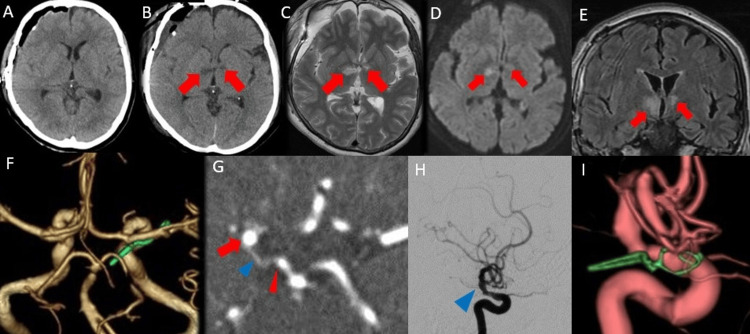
Postoperative imaging findings. (A) Non-contrast computed tomography (CT) obtained on postoperative day one, axial view, showing no apparent abnormality in the brain parenchyma. (B) Non-contrast CT obtained on postoperative day three, axial view, demonstrating low-density areas in the bilateral ventral thalami (arrows). (C) T2-weighted magnetic resonance imaging obtained on postoperative day 11, axial view, demonstrating infarcts in the same bilateral ventral thalamic regions. (D) Diffusion-weighted imaging obtained on postoperative day 11, demonstrating bilateral diffusion-restricted lesions corresponding to the abnormalities seen on the T2-weighted and fluid-attenuated inversion recovery images. (E) Fluid-attenuated inversion recovery magnetic resonance imaging obtained on postoperative day 11, coronal view, demonstrating infarcts in the same bilateral ventral thalamic regions. (F) Postoperative three-dimensional computed tomography angiography (CTA), superior view, showing that the tip of the clip does not extend to the contralateral side. (G) Postoperative three-dimensional CTA, axial view, showing the posterior communicating artery (blue arrowhead) arising posteriorly from the internal carotid artery (red arrow), as in the preoperative study. The P1 segment is also well visualized (red arrowhead). (H) Digital subtraction angiography obtained on postoperative day 17, lateral view, clearly demonstrating the posterior communicating artery (blue arrowhead). (I) Three-dimensional rotational angiography reconstructed image obtained from the same angiographic study, in which the posterior communicating artery is not clearly depicted on the three-dimensional reconstruction.
